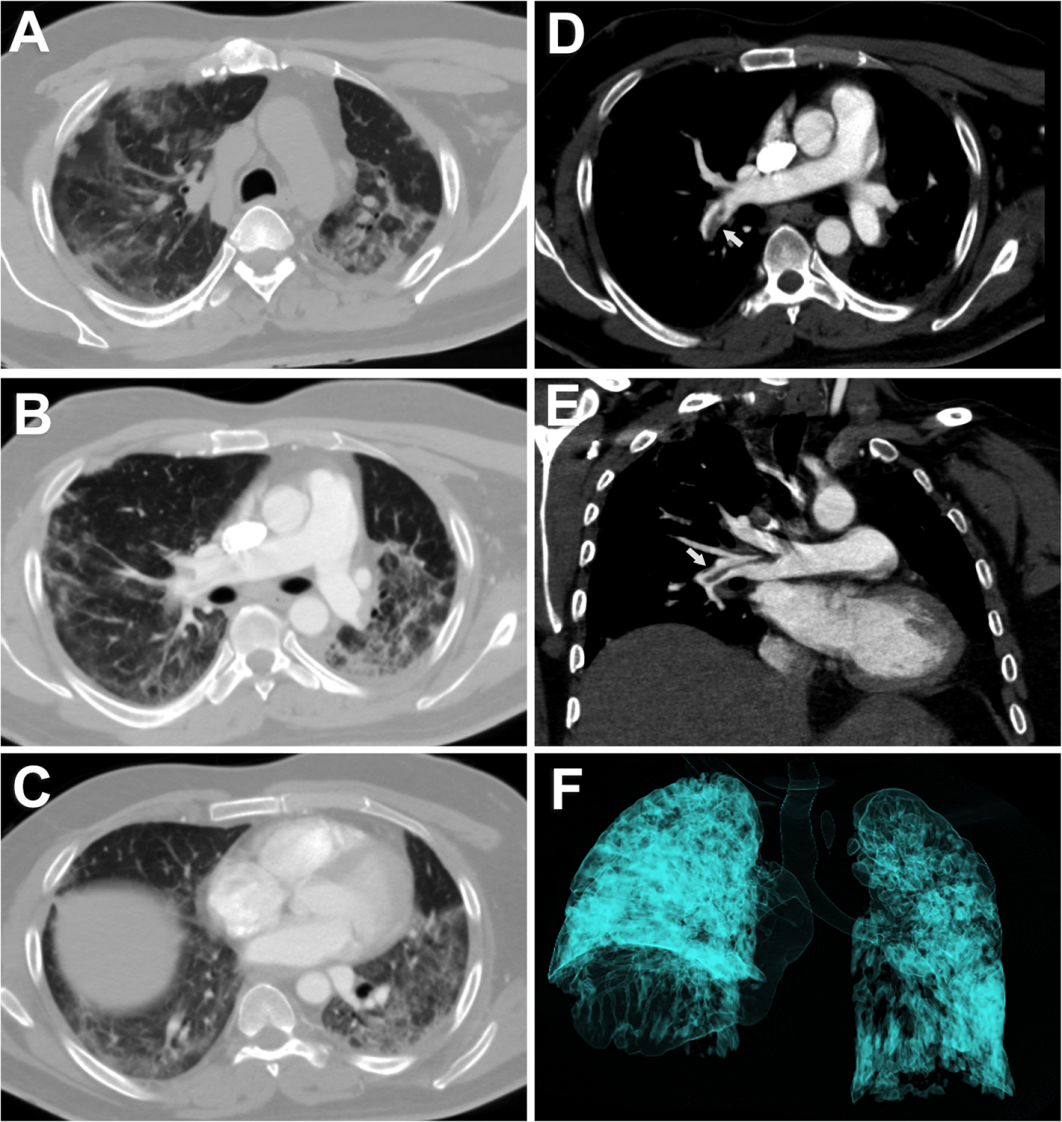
Non-contrast axial CT of the chest of a 45-year-old male patient with positive RT-PCR. Bilateral ground-glass opacities are seen of mainly peripheral distribution (a–c). The patient had a high D-dimer level. Thus, CT pulmonary angiography was done (d, e), revealing right descending pulmonary arterial thromboembolism (arrows). 3D reconstruction volume rendering image shows pulmonary involvement as opaque non-transparent shadows (f). CT severity score is 15 points which corresponds to moderate involvement. The patient received anticoagulant treatment and improved on follow-up
